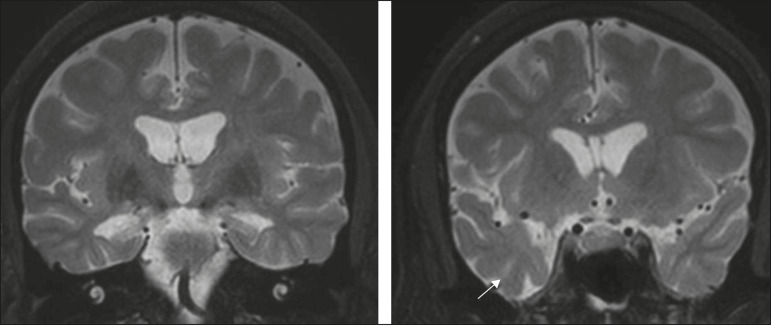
A 62-year-old male patient with refractory seizures. Coronal T2-weighted MRI sequence showing a reduction in the volume of both hippocampi, with a hyperintense signal and a loss of rugosity on the upper surface (cytoarchitectural alteration). Note the blurring of the transition between the gray and white matter in the right temporal pole (arrow).
